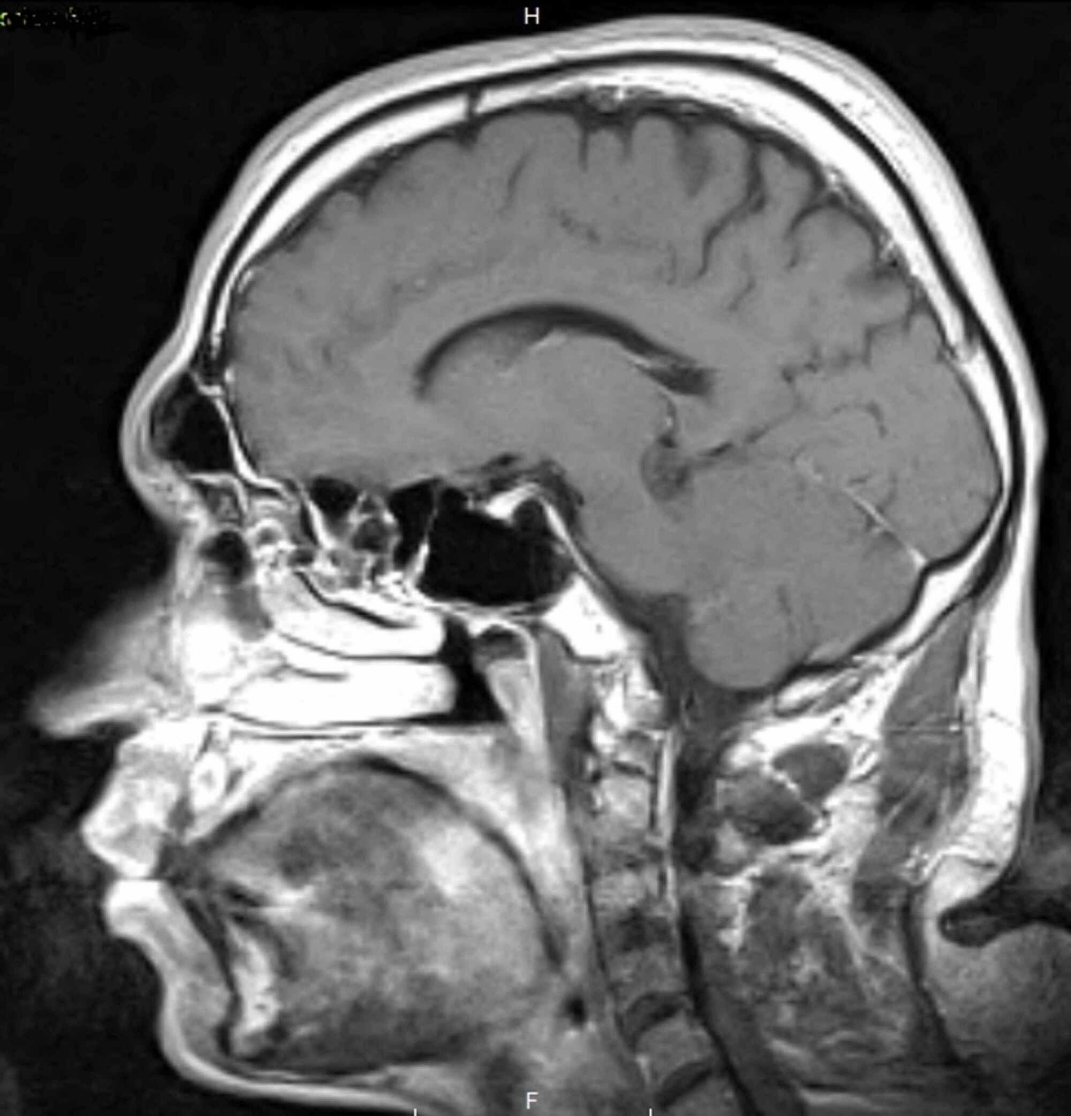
A sagittal plane MRI of the patient’s brain.
